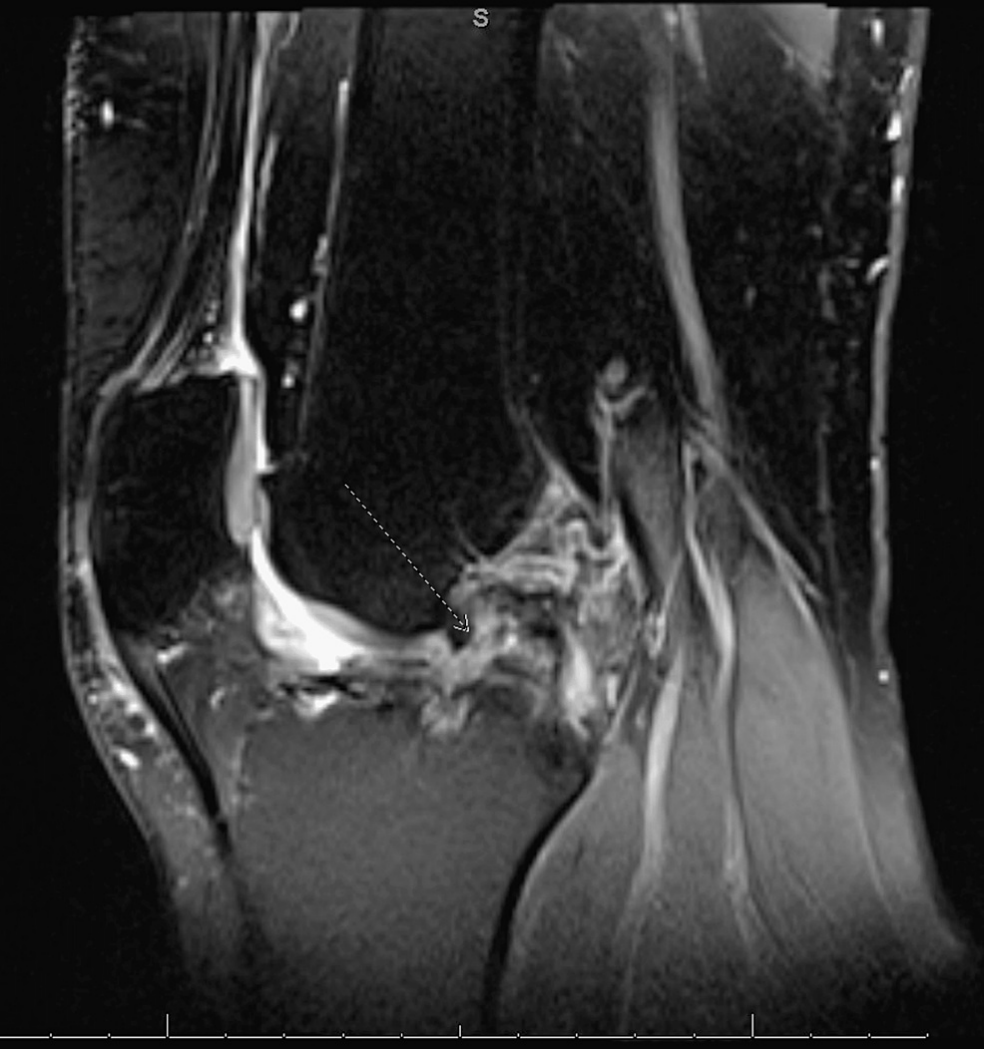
MRI of left knee, lateral view showing previous ACL graft with signal changes concerning for a graft tear (arrow). ACL: anterior cruciate ligament
